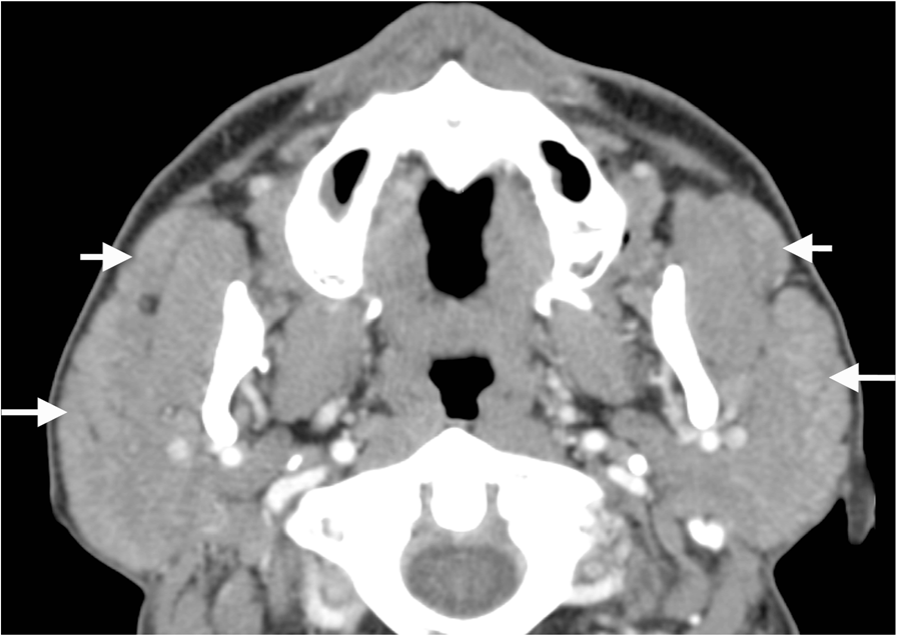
Bilateral enlargement of the accessory parotid glands (*short arrows*) and main parotid glands (*long arrows*) are observed on CT film
